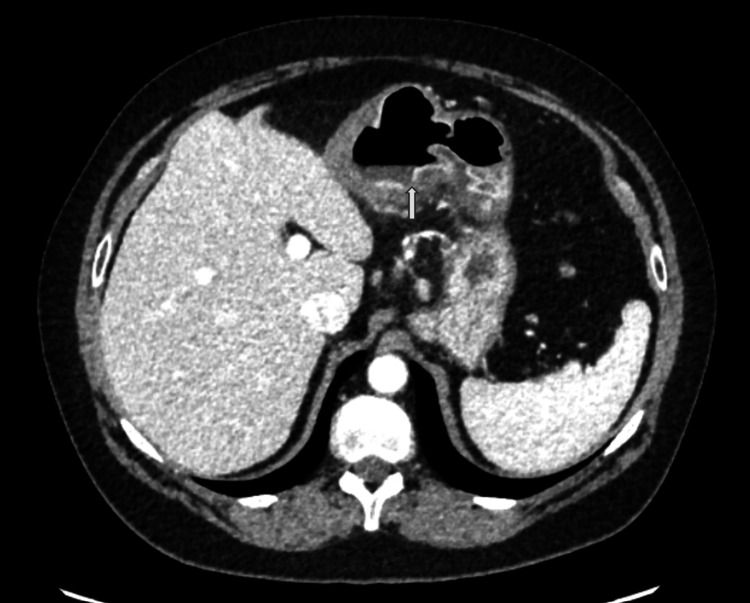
Axial CT of the abdomen and pelvis with contrast: a low-density discontinuity in the mucosa of the gastric antrum is seen (white arrow), which is suggestive of a gastric ulcer in this patient’s presentation. CT: computed tomography
